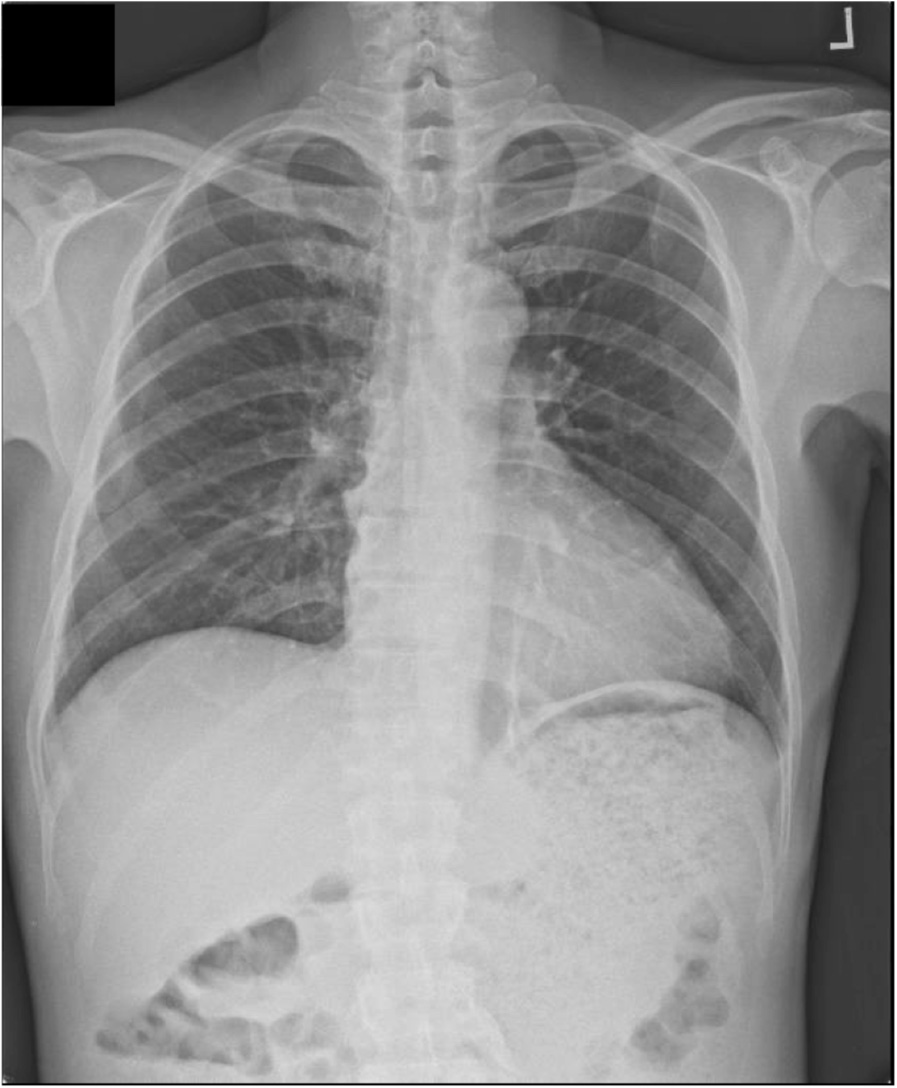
Chest X-ray showing distended gastric outline with food residue
